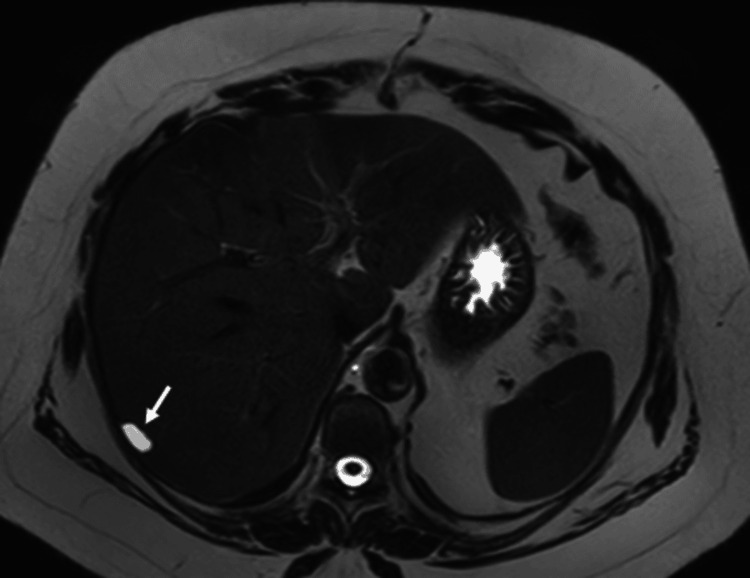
Abdominal MRI (liver cyst). Short-TI inversion recovery (STIR) abdominal MRI scan shows a hypervascular lesion in segment VII of the right liver lobe, measuring about 1.6 cm, retaining IV contrast (white arrow).
